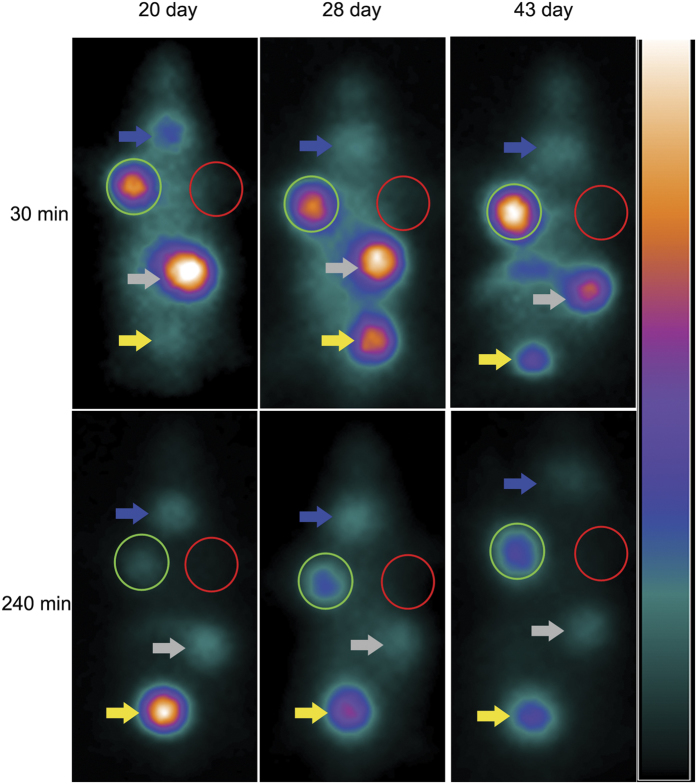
Three imaging at different days after transplantation with screened Bac-NIS-SB-NeoR-U87 cells by SPECT. The successfully detecting of Bac-NIS-SB-NeoR-U87 tumor cells at 30 minutes in each SPECT imaging *in vivo*. The green and red circle respectively represent the Bac-NIS-SB-NeoR-U87 cells and U87 cells. Blue, gray and yellow arrows respectively represent thyroid, stomach and bladder.
